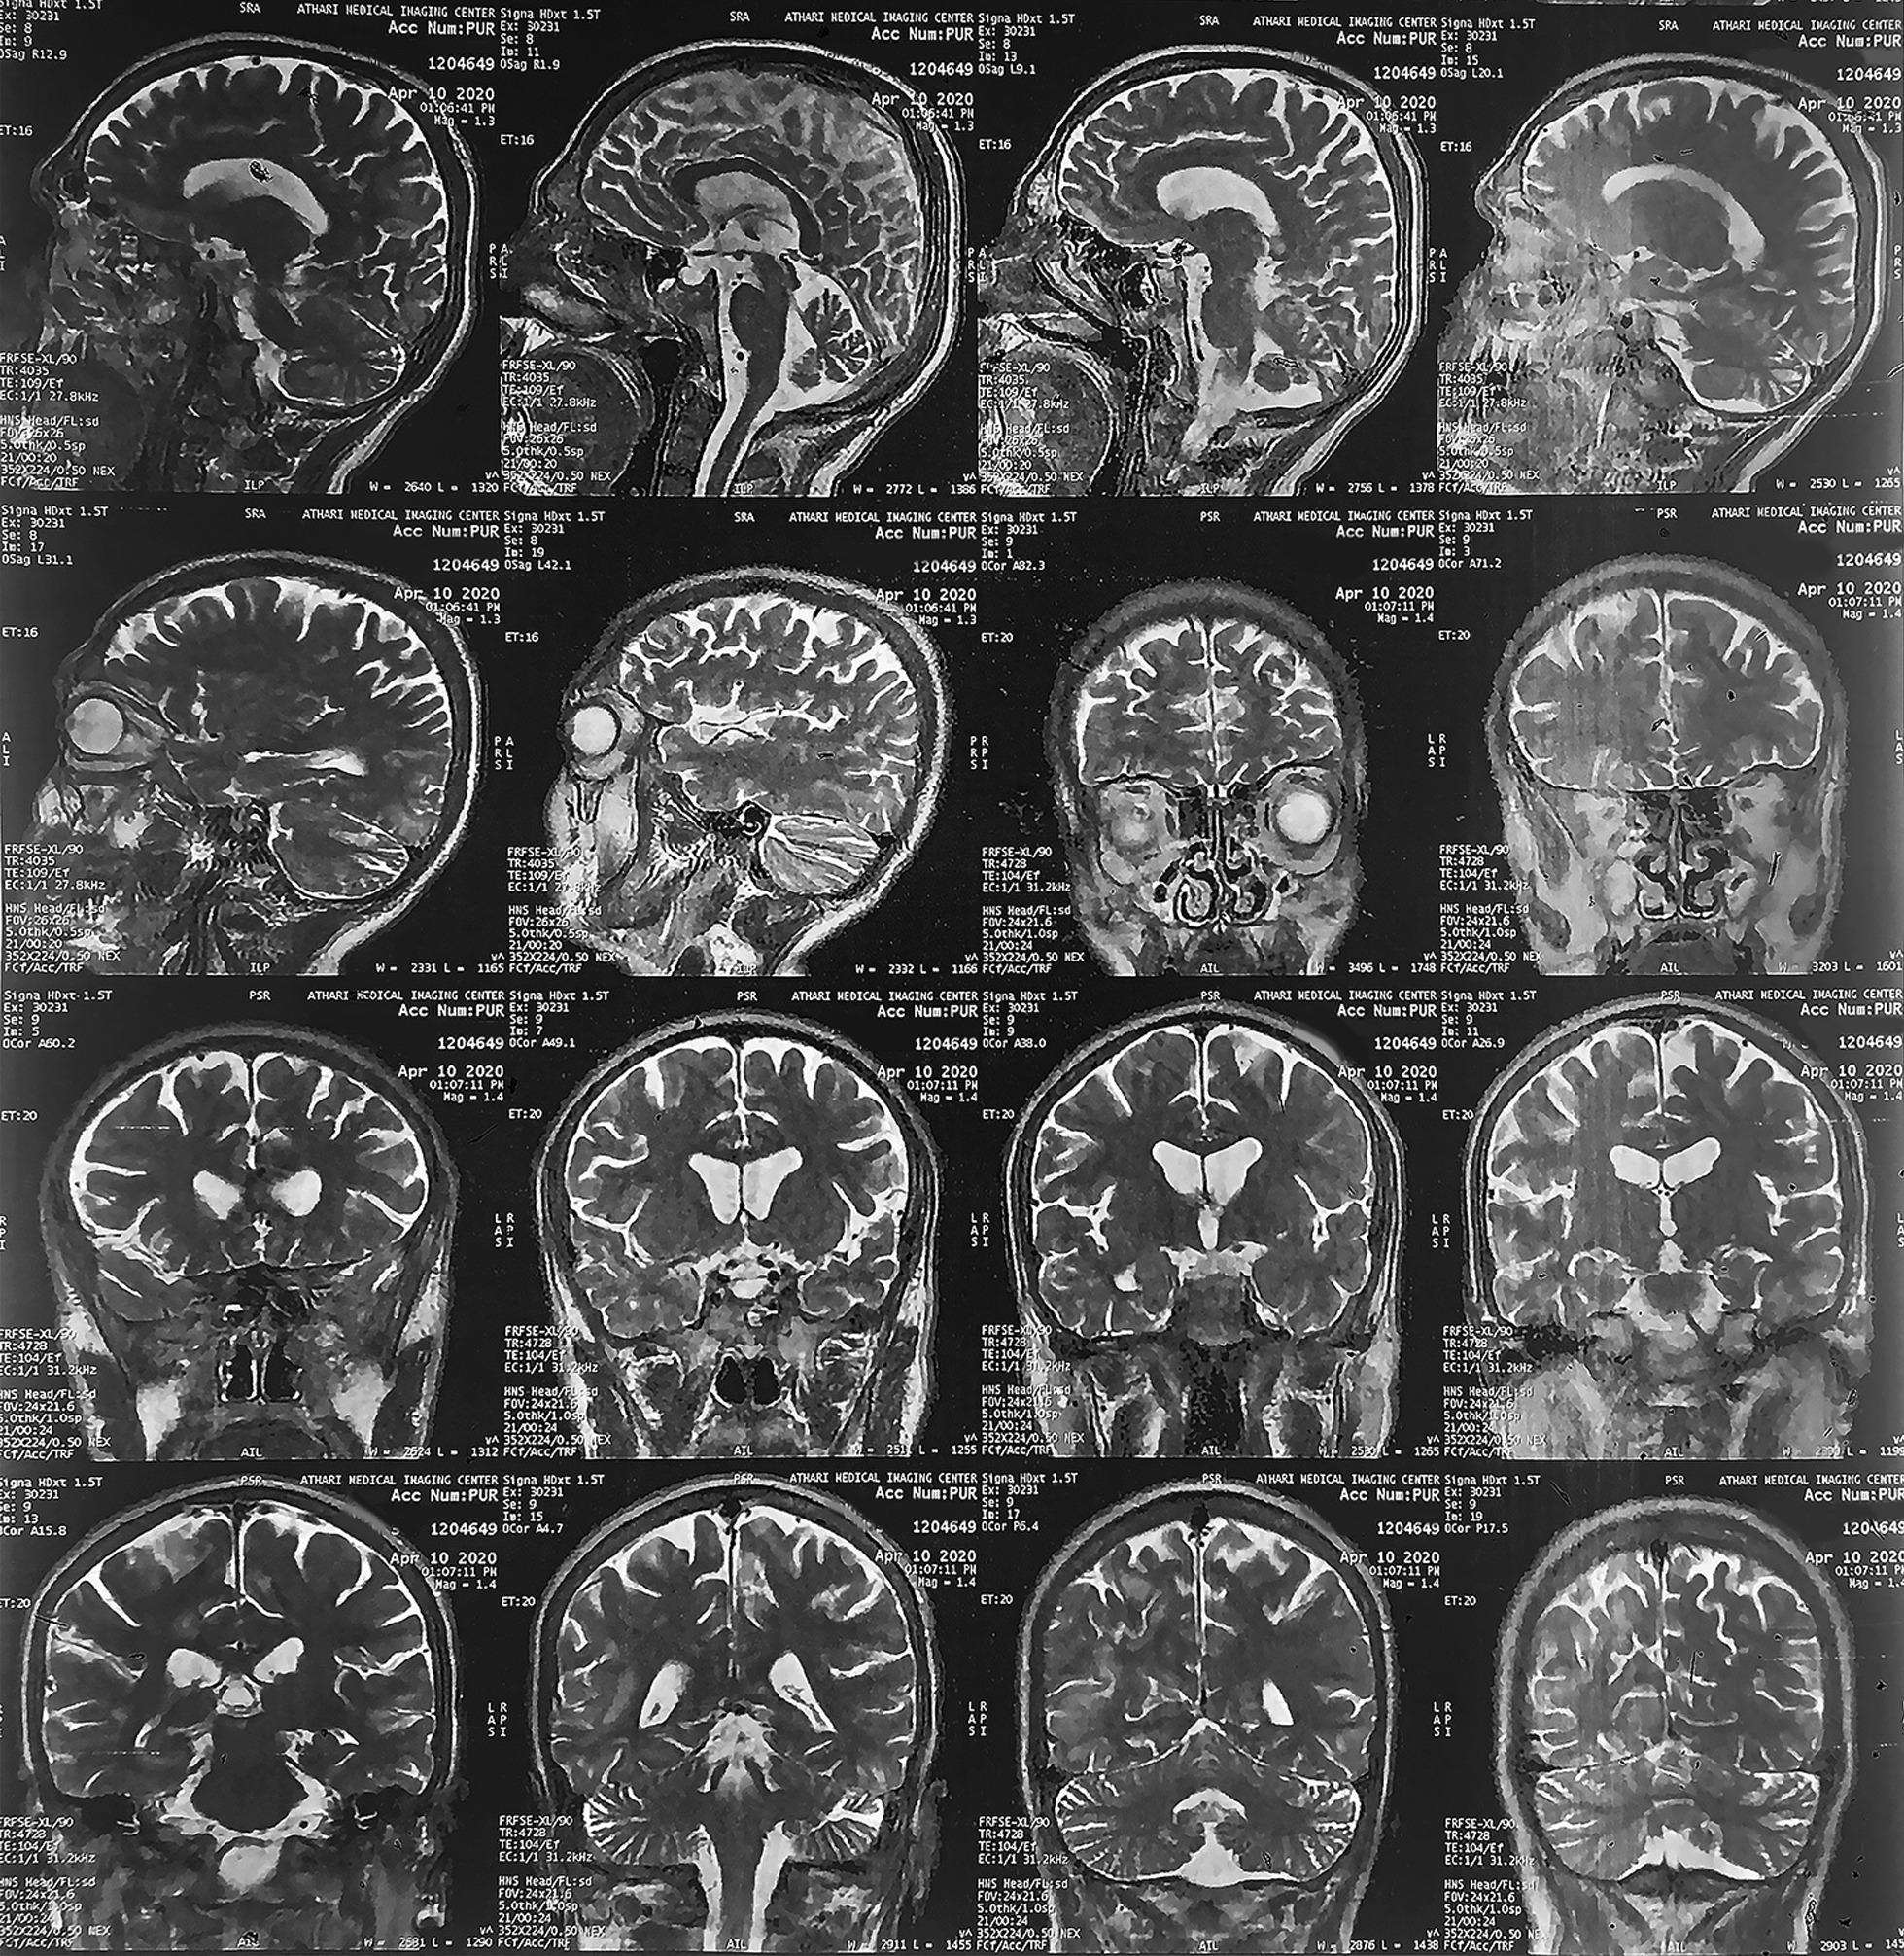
Magnetic resonance imaging of the brain, indicting subcortical signal changes in the left side of the splenium corpus callosum and the right side of the thalamus
